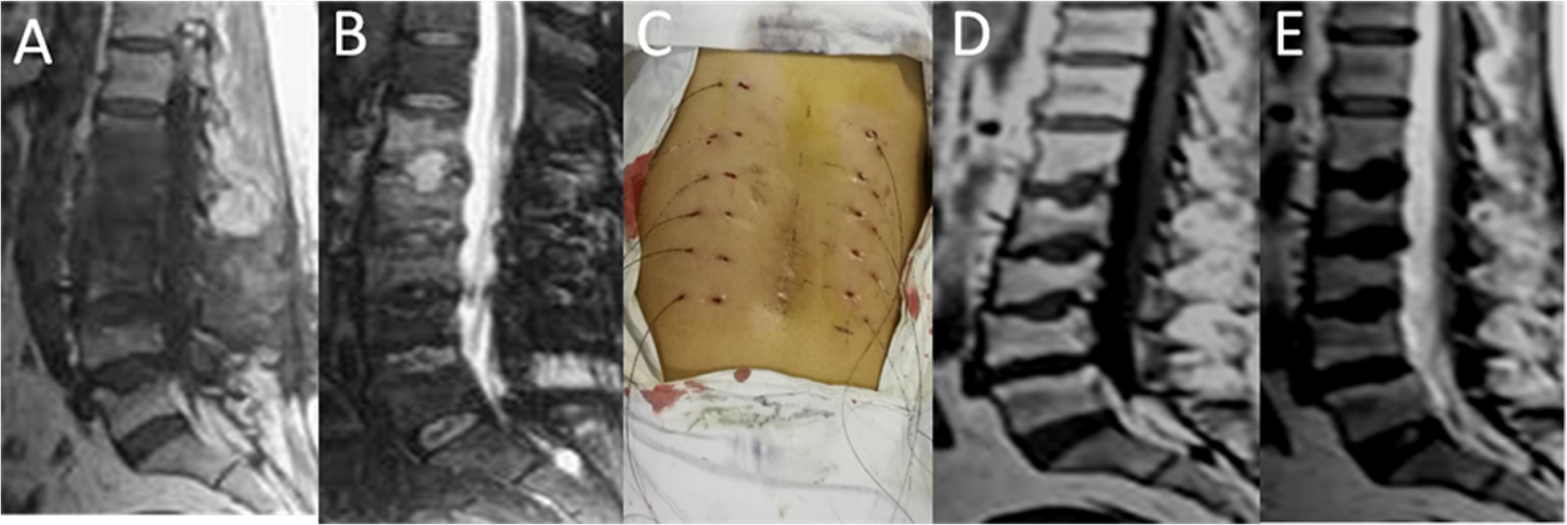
The same 49-year-old female as shown in Fig. 4 (case 2). Lesion debridement + fixation removal was performed. MRI in our institution showed that the infection had progressed to the L1/L2 level, with endplate destruction six weeks after fixation removal (a) and (b). Clinical photograph of postoperative percutaneous debridement and irrigation (c). At 12-month follow-up, MRI T1(d) and T2(e) showed all infection had resolved
